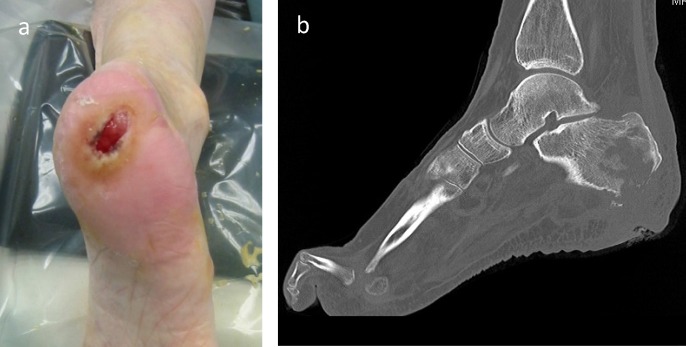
2a: Plantar ulceration over calcaneal osteomyelitis in a patient with paraplegia after spinal cord injury. 2b: CT Scan showing the bone destruction deep to the ulcer.
